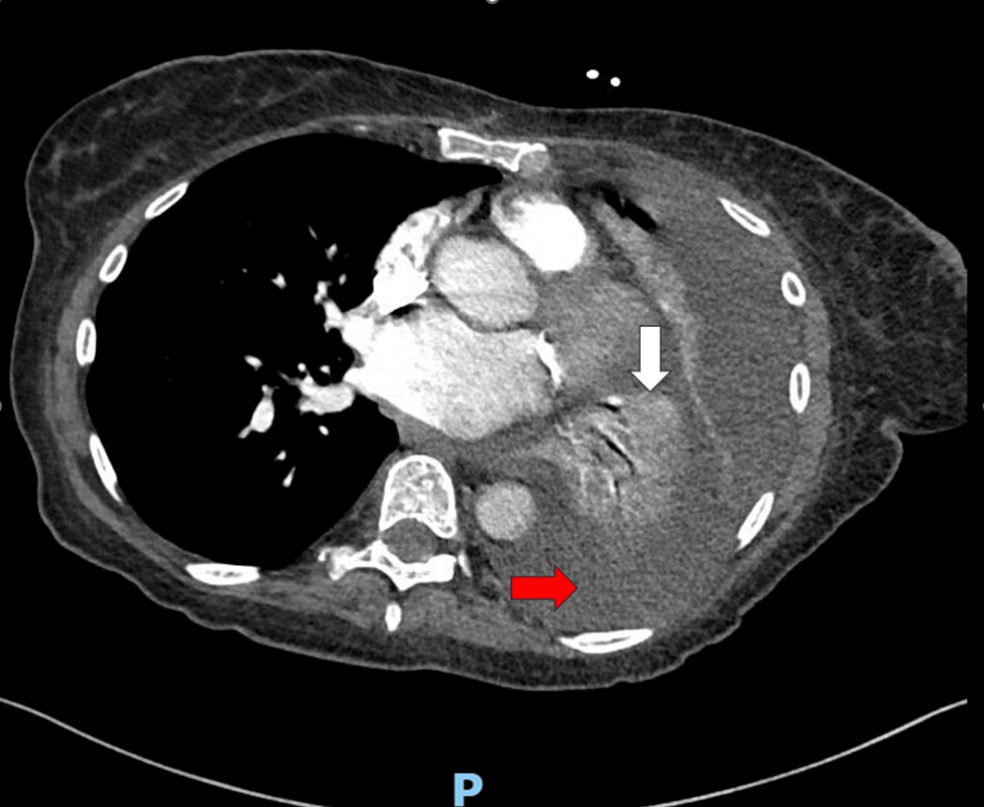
CT scan of the chest (mediastinal window) showing left pleural effusion (red arrow) with collapse of the left lower lobe (white arrow)
